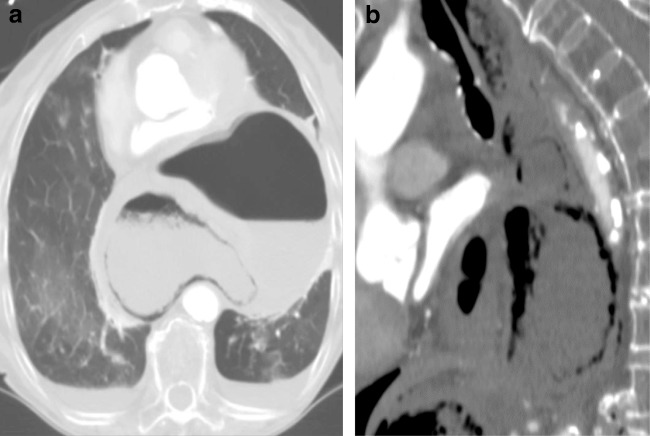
a, b A 92-year-old female with acute onset of severe upper abdominal pain, vomiting and nausea. Sagittal (a) and axial (b) contrast CT of the thorax (double rule out) demonstrated an intrathoracic stomach with organoaxial volvulus and gastric pneumatosis (Movie 3) requiring emergent surgical repair
